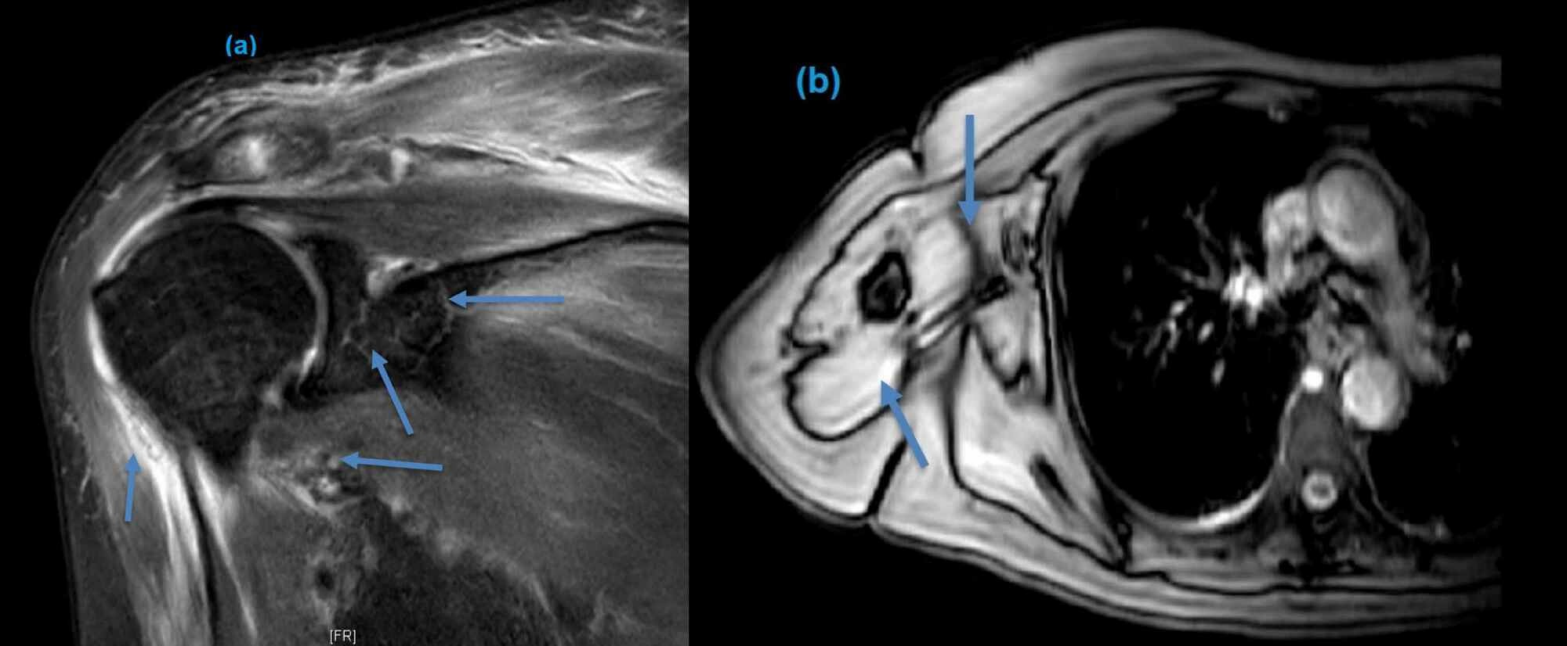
Magnetic resonance imaging (MRI) of the right shoulder showing joint effusion extending up to sub-coracoid space and proximal humerus associated with soft tissue edema (blue arrow) in coronal (a) and axial (b) view.
